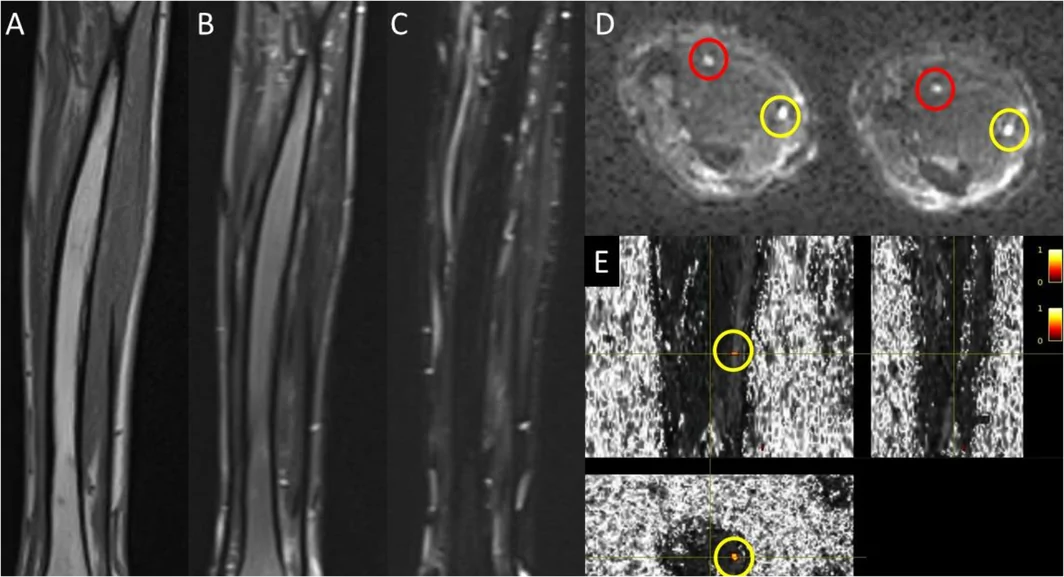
MR Neurography image acquisition. Coronal MR imaging of the right forearm (A: T1‐weighted Vibe Dixon, B: T2‐weighted, C: T2‐STIR‐weighted). (D) Axial DTI of the distal forearm allowing for the identification of median (red circles) and ulnar (yellow circles) nerves. (E) Region of interest drawn on right ulnar nerve (yellow circles) to be used to seed tractography.
